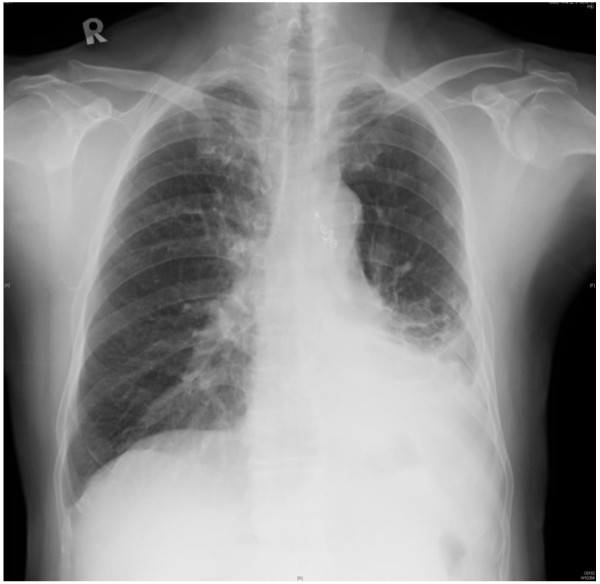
At 10 months postoperatively chest plain film sowed no progression in left lower lobe opacity.
